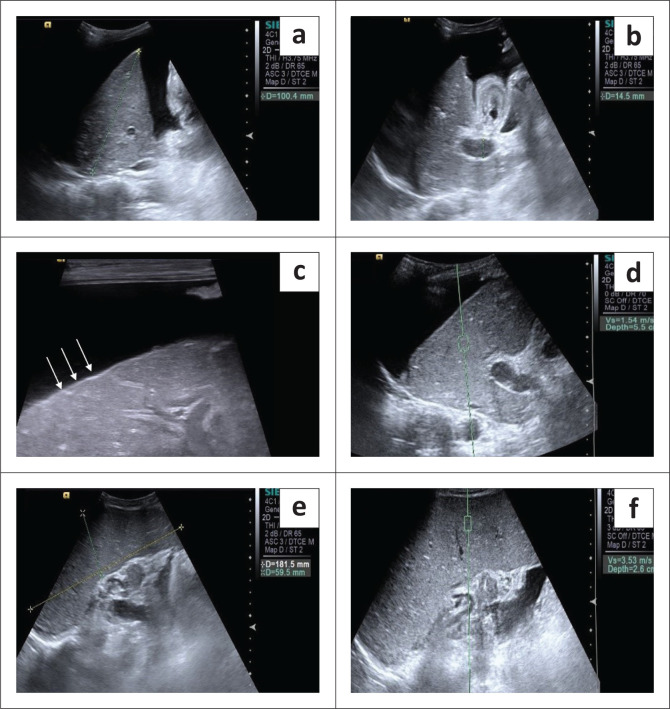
A 40-year-old female with chronic liver disease and large oesophageal varices: Grey scale ultrasound reveals gross ascites and an altered hepatic echo-pattern (a) with a dilated portal vein, measuring 14.5 mm at the porta and an oedematous gall bladder wall (b). Evaluation with the high frequency probe better depicts the hepatic surface nodularity (arrows) (c) Hepatic shear wave velocity (SWV) measures 1.54 m/s (d). Splenomegaly with normal echo texture (e). Splenic acoustic radiation force imaging measurement with ROI placed about 2.6 cm below the skin surface shows SWV in this case to be 3.53 m/s (f).
